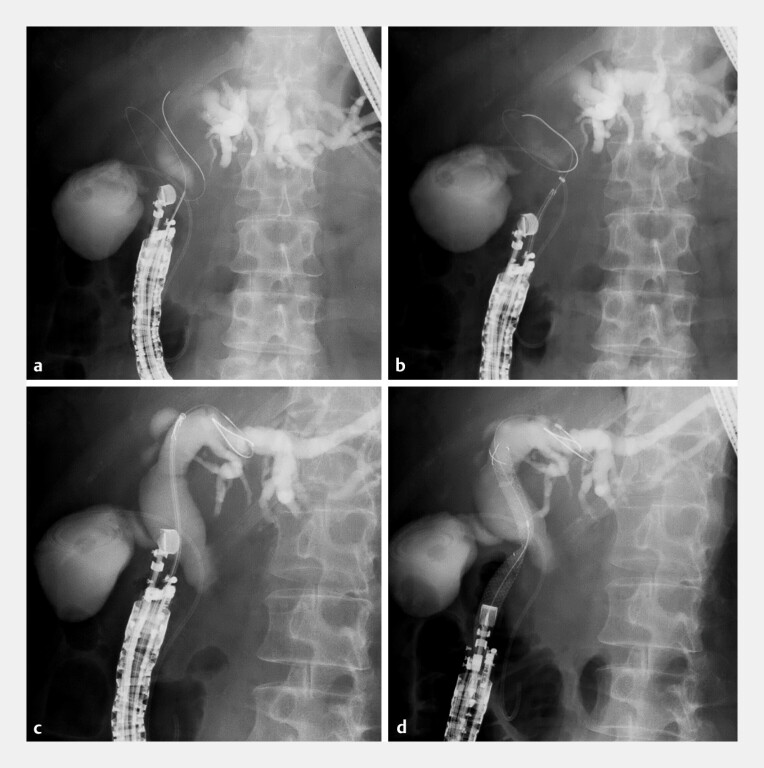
Endoscopic ultrasonography-guided choledochoduodenostomy.
